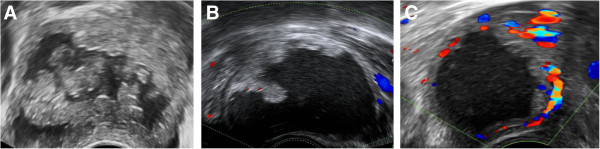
Sonographic appearances of decidualised endometrioma. Unilocular cysts containing hyperechoic fluid and irregular internal wall with prominent echogenic rounded papillary projections [A]. The papillary projections were typically highly vascular on Doppler examination [B and C].
